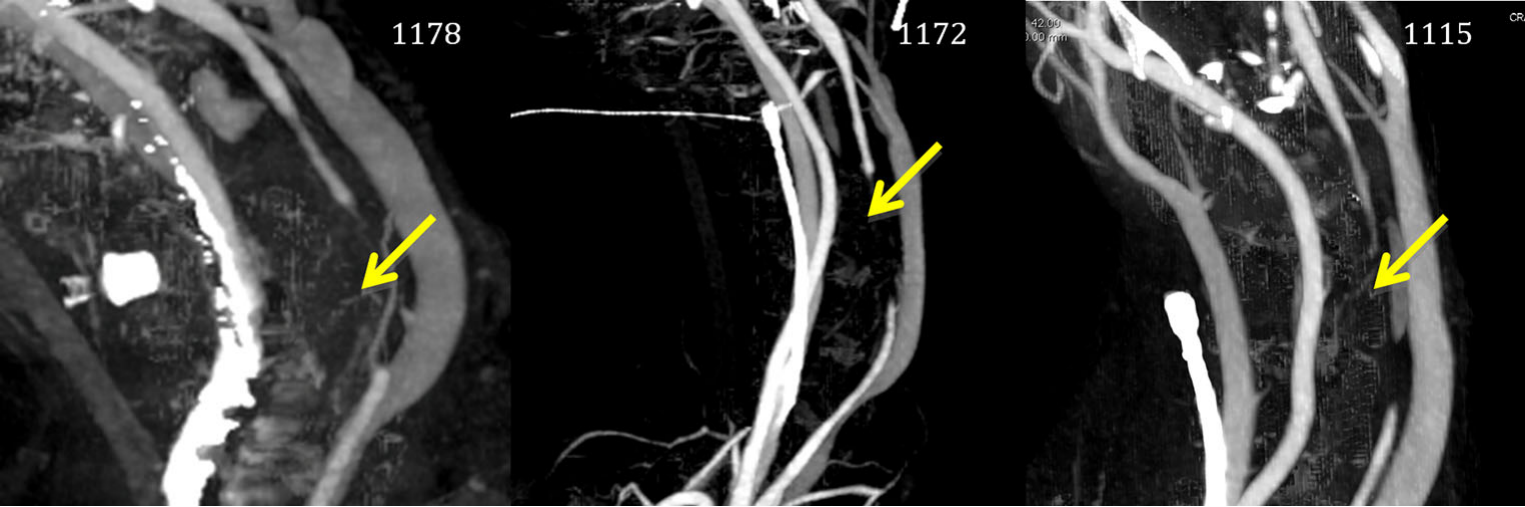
CT images of the occluded carotid artery
